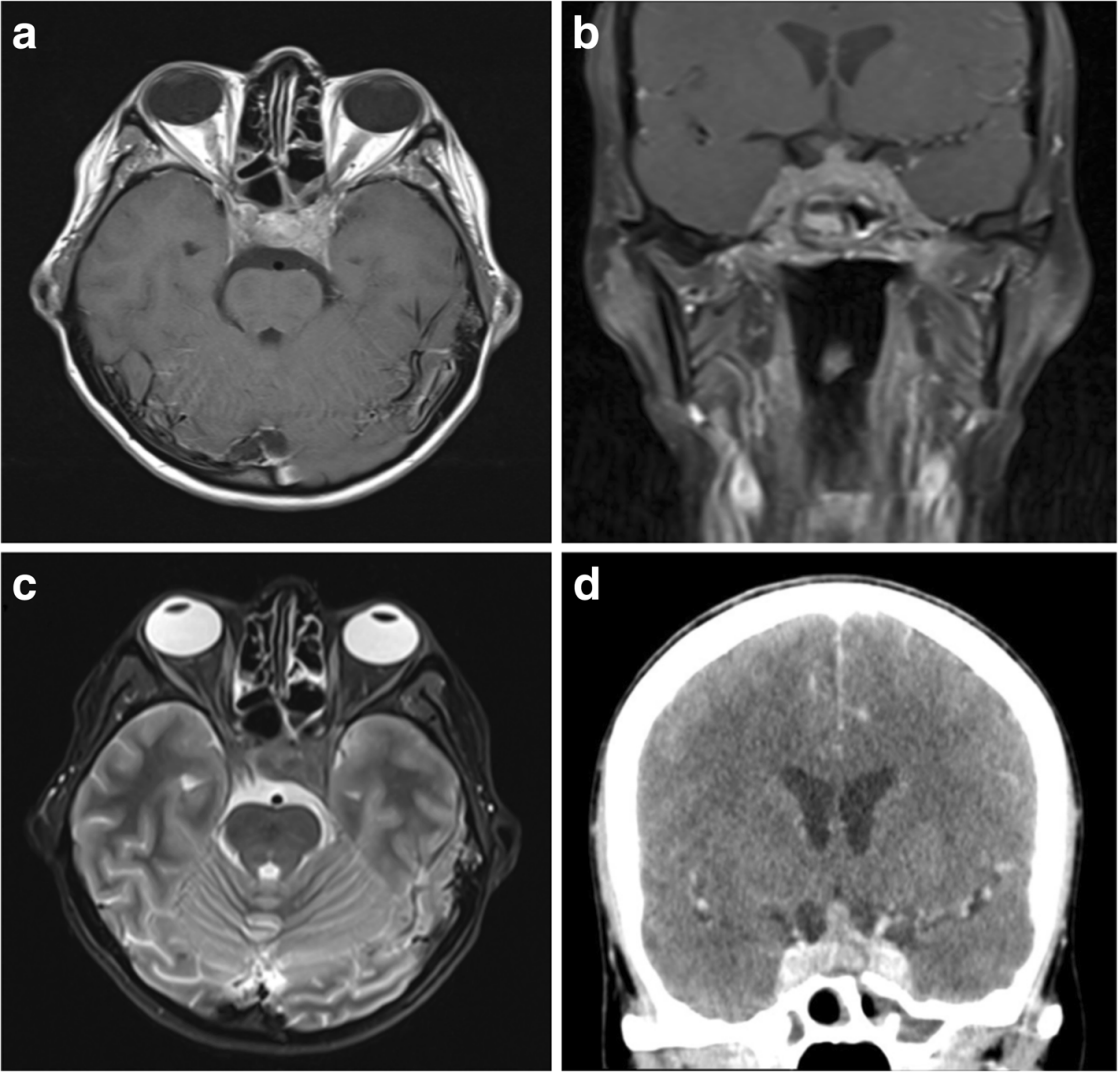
Granulomatosis with polyangiitis of the skull base. a, b Post-contrast T1-weighted axial and coronal MR images showing enhancing inflammatory soft tissue involving the skull base and cavernous sinuses. There is also inflammatory change within the adjacent sphenoid sinus. c T2-weighted axial image of the same lesion at the level of the cavernous sinuses. d Post-contrast axial CT image again showing avid enhancement within durally based abnormal soft tissue
